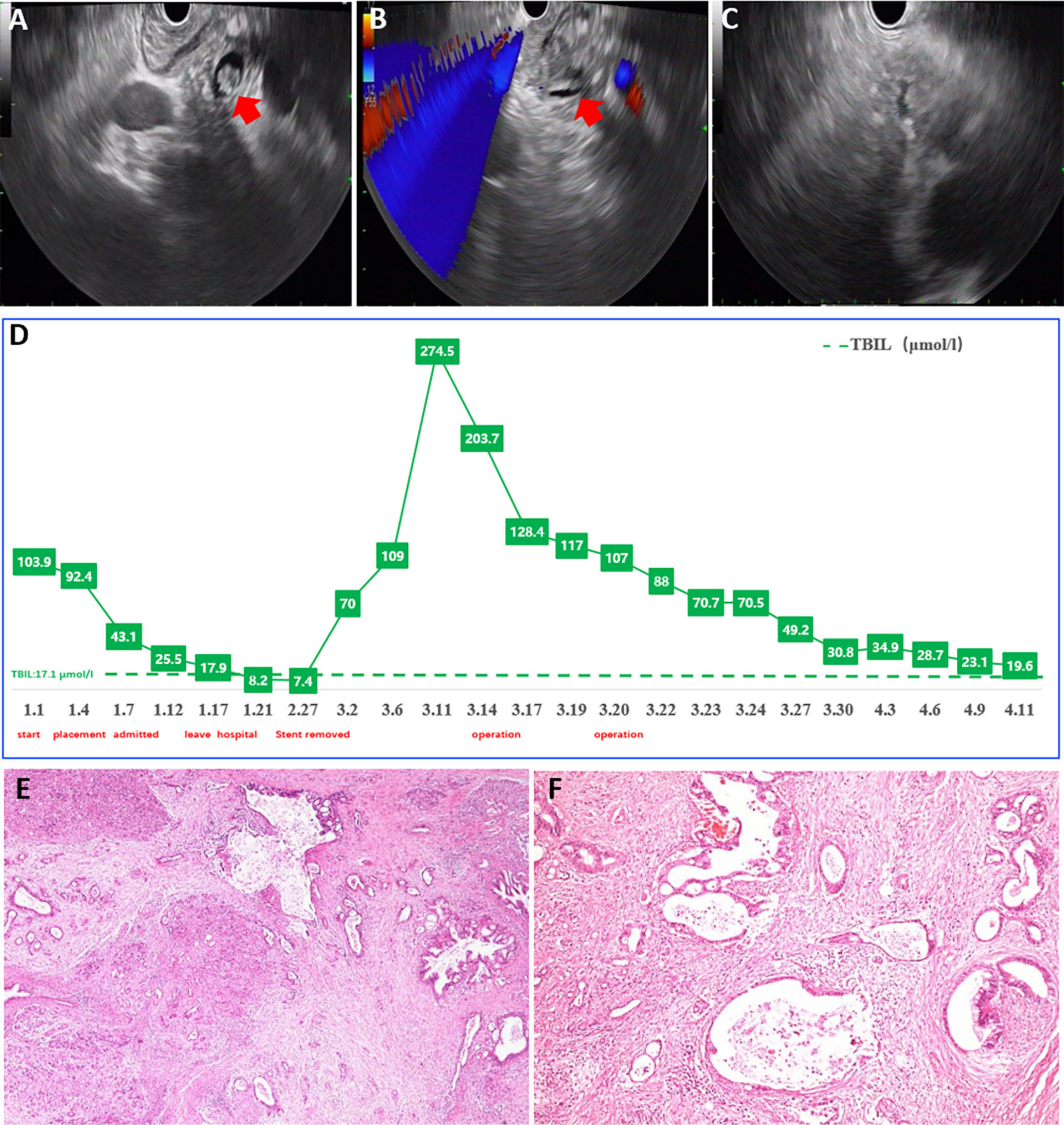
Examination results after readmission. (A–C) EUS confirmed a lesion in lower common bile duct. (D) The serum level of TBIL after pancreatoduodenectomy. (E, F) Pathology evaluation confirmed the diagnosis as moderately differentiated adenocarcinoma of common bile duct. EUS, endoscopic ultrasonography; TBIL, total bilirubin.
